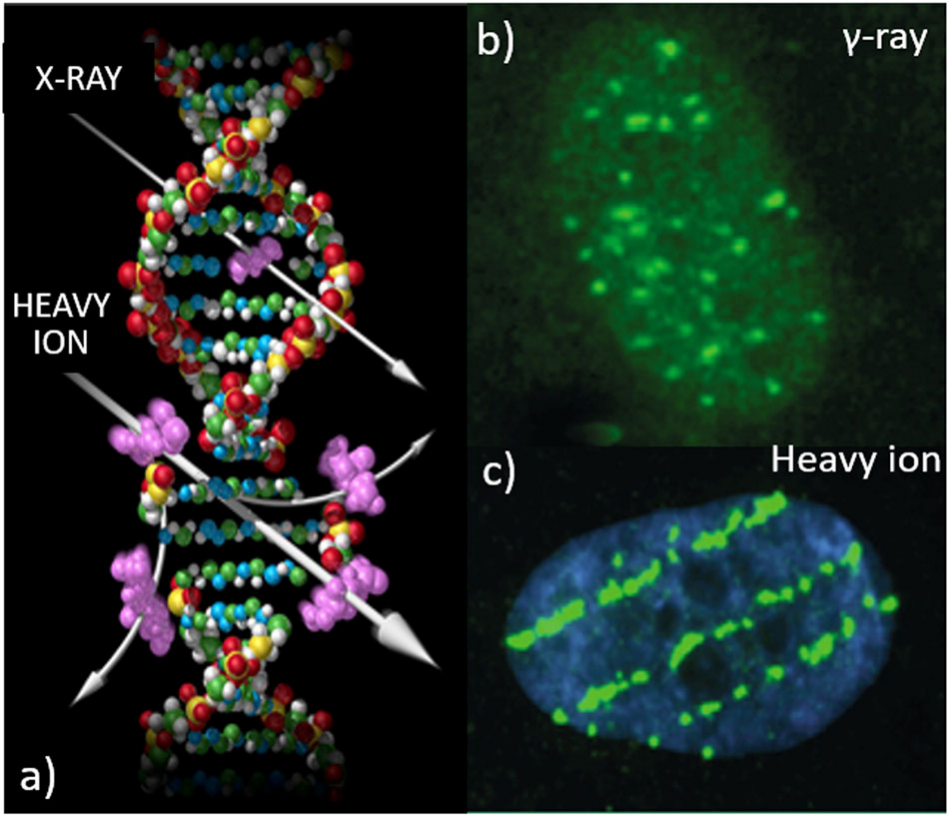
Galactic cosmic rays are qualitatively different from X-rays or gamma-rays. a HZE ions produce dense ionization along the particle track as they traverse a tissue and impart distinct patterns of DNA damage compared to terrestrial radiation such as X-rays. γH2AX foci (green) illuminate distinct patterns of DNA double-strand breaks in nuclei of human fibroblast cells after exposure to b gamma-rays, with diffuse damage, and c HZE ions with single tracks. Image credits: NASA (a) and Cucinotta and Durante^97^ (b and c).
